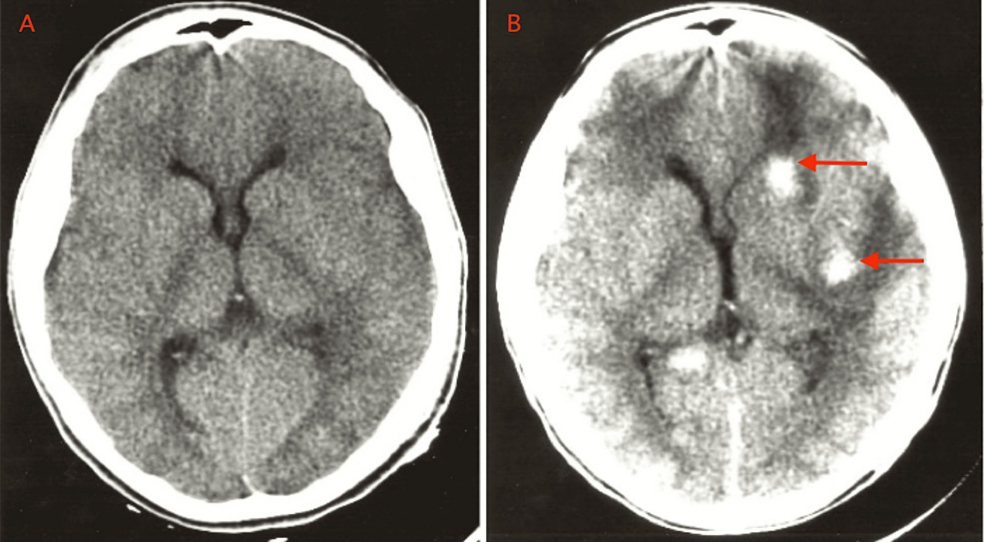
CT images for a patient with DTICH type 3 CT images for a patient experiencing DTICH type 3 are demonstrated. The left image (panel A) shows the initially negative CT scan. The patient had other traumatic injuries and was taken to the operating room. The right image (panel B) demonstrates the resulting CT scan following post-operative neurologic changes. The patient was found to have de novo hematoma (red arrows in panel B) in the follow-up head CT. CT: computed tomography; DTICH: delayed traumatic intracerebral hematoma
